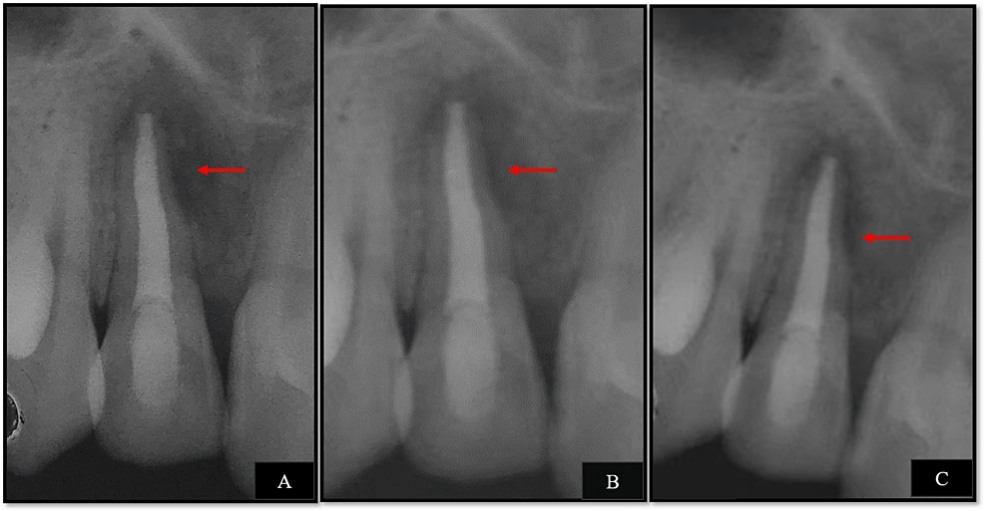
Follow-up radiographs (A) Three months follow-up, (B) six months follow-up, and (C) nine months follow-up
